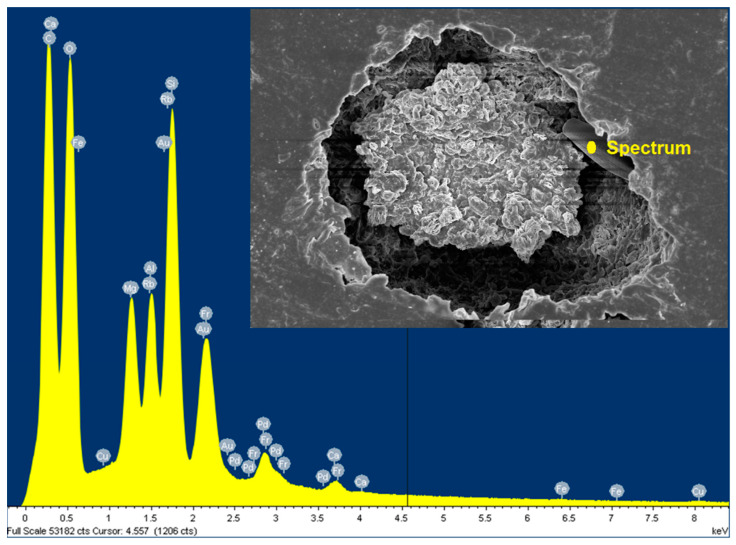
SEM energy dispersive X-ray analysis (SEM-EDX) analysis of PBS/St-Mg Al LDH after 10 weeks of seawater immersion.
